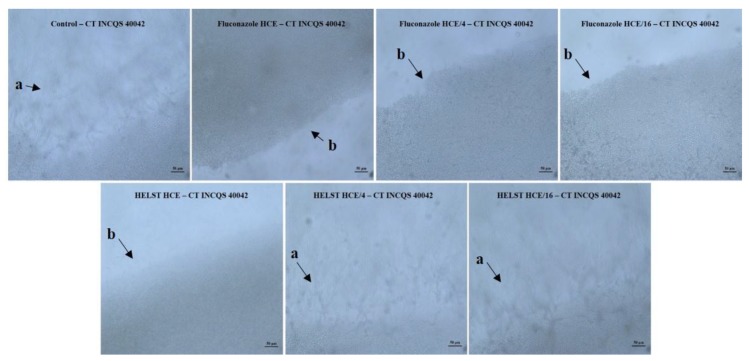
Demonstrative effect of HELST and fluconazole on the morphological transition of *Candida tropicalis*. CT: *Candida tropicalis*; INCQS: National Institute of Quality Control in Health; URM: University Recife Mycology; HELST: Hydroalcoholic Extract of Leaves of *Spondias tuberosa*; FCZ: Fluconazole; HCE: Higher Concentration Evaluate; a: presence of filamentous structures; b: absence of filamentous structures. A—hyphae development; B—hyphae inhibition.
